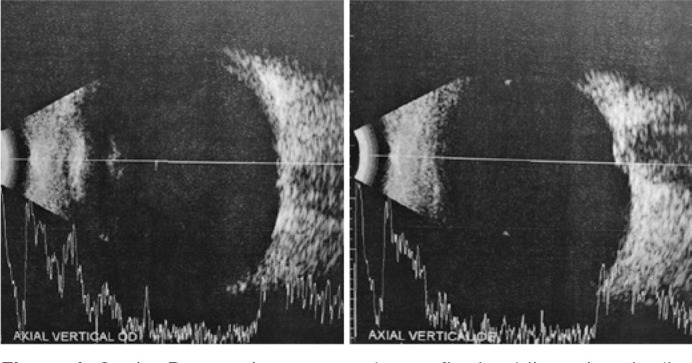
Ocular B-scan ultrasonography confirming bilateral optic disc drusen.
